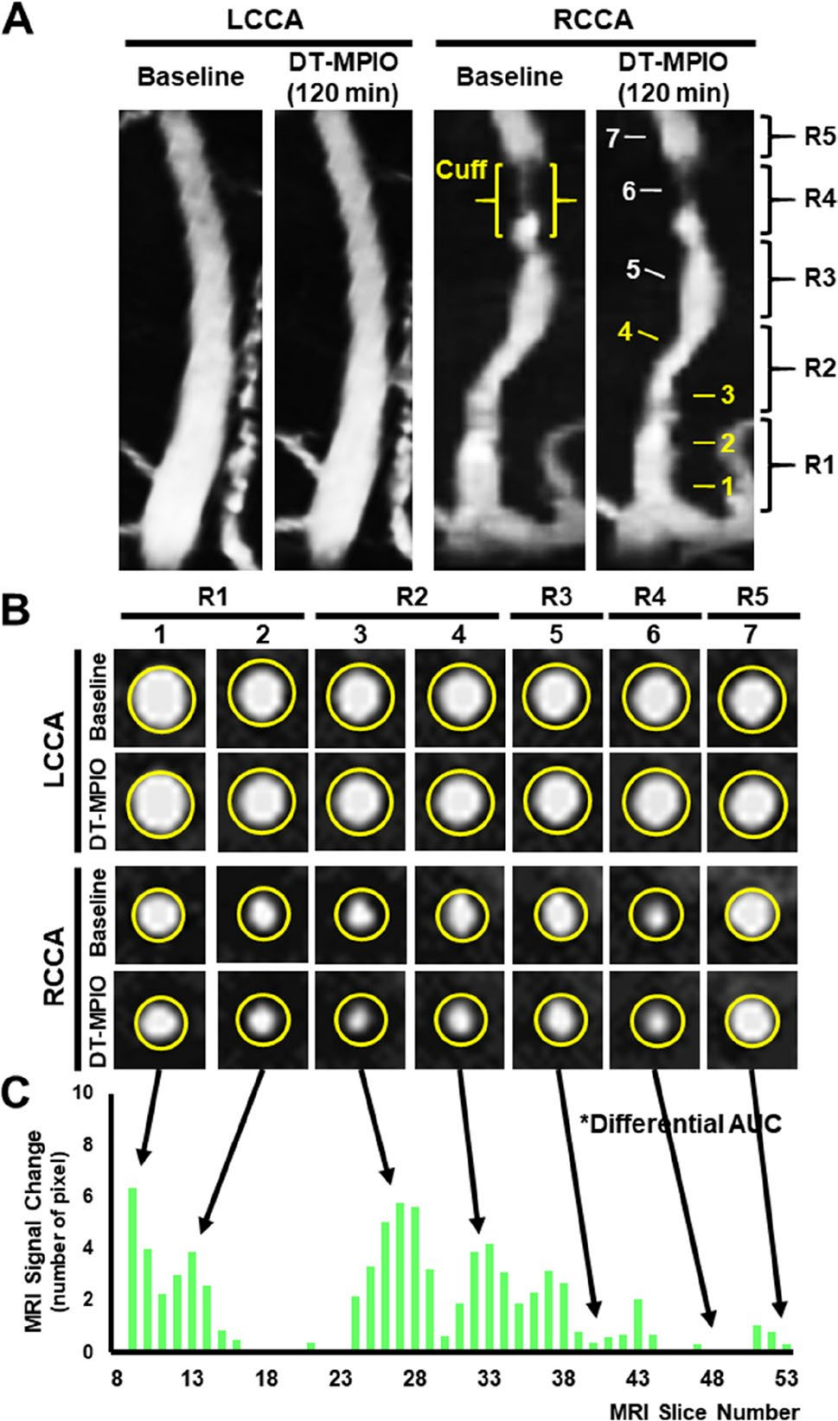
Quantification of magnitude of MR signal change. (A) The pre- and post-contrast 3D-TOF MRA images of RCCA and LCCA. (B) Axial MR images of RCCA and LCCA. New areas of hypointense signal were identified on the respective axial slices in R1–3 of RCCA. No new hypointense signal was detected on the axial slices in R4 and R5 of RCCA, and throughout LCCA in the post-contrast images. (C) Quantification of magnitude of MR signal change between the pre- and post-contrast carotid images. The magnitude of signal change was greater in R1–3 of RCCA than R4 or R5
